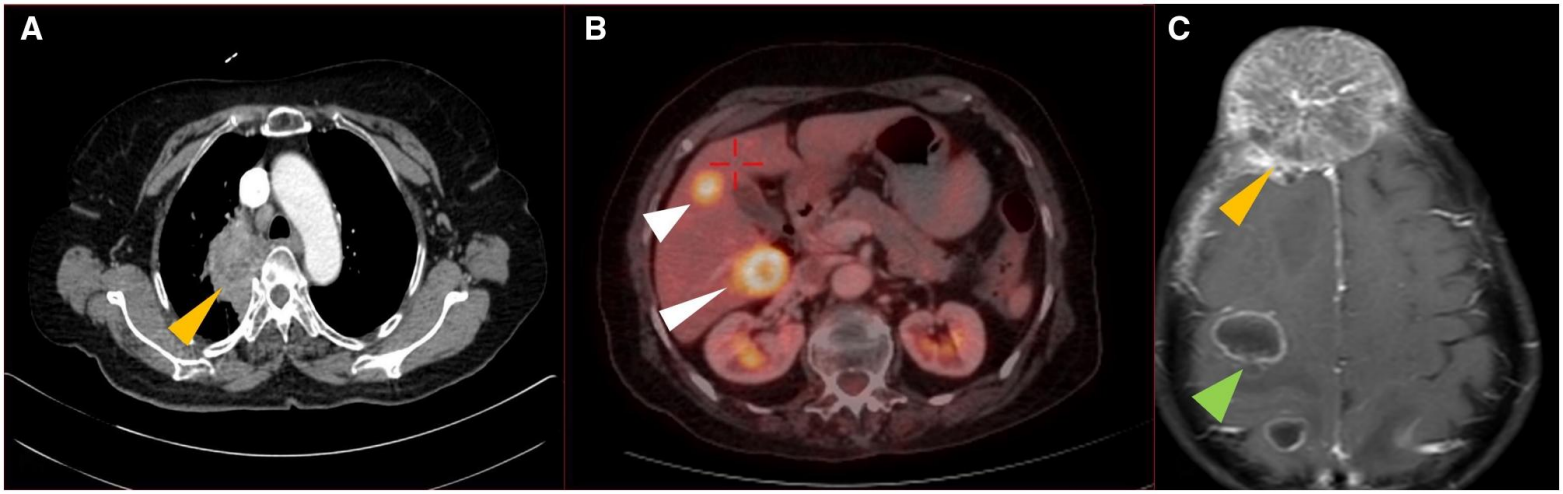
62 years old male with stage IVB squamous cell carcinoma. (A) Right perihilar mass (arrowhead) infiltrating the mediastinum with metastatic level 2R node. (B) Liver metastases (arrowheads) showing avidity on fluorodeoxyglucose positron emission tomography computed tomography (FDG PET/CECT). (C) MRI brain showing brain parenchymal metastasis in right precentral gyrus (green arrowhead) and frontal bone calvarial metastasis (yellow arrowhead).
